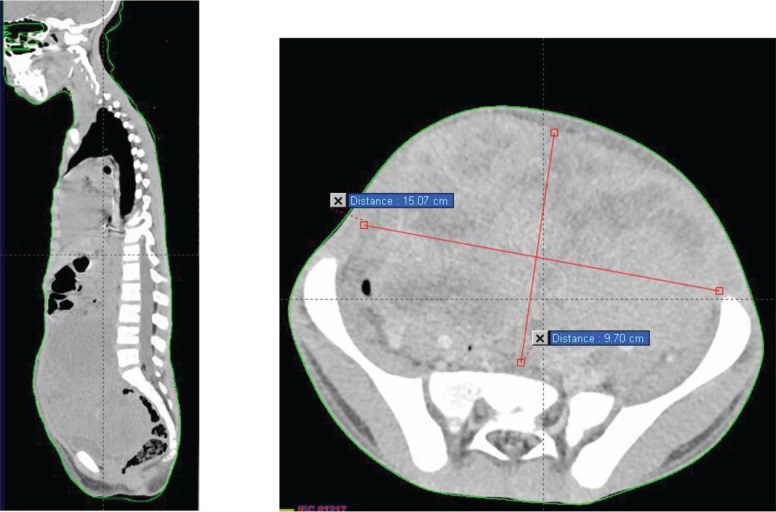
Chest–abdomen–pelvis prechemo CT scan showing a voluminous abdominal–pelvic compressive massively necrotic vascularized tumour process, responsible for compression of the adjacent digestive structures laterally and inferiorly.
